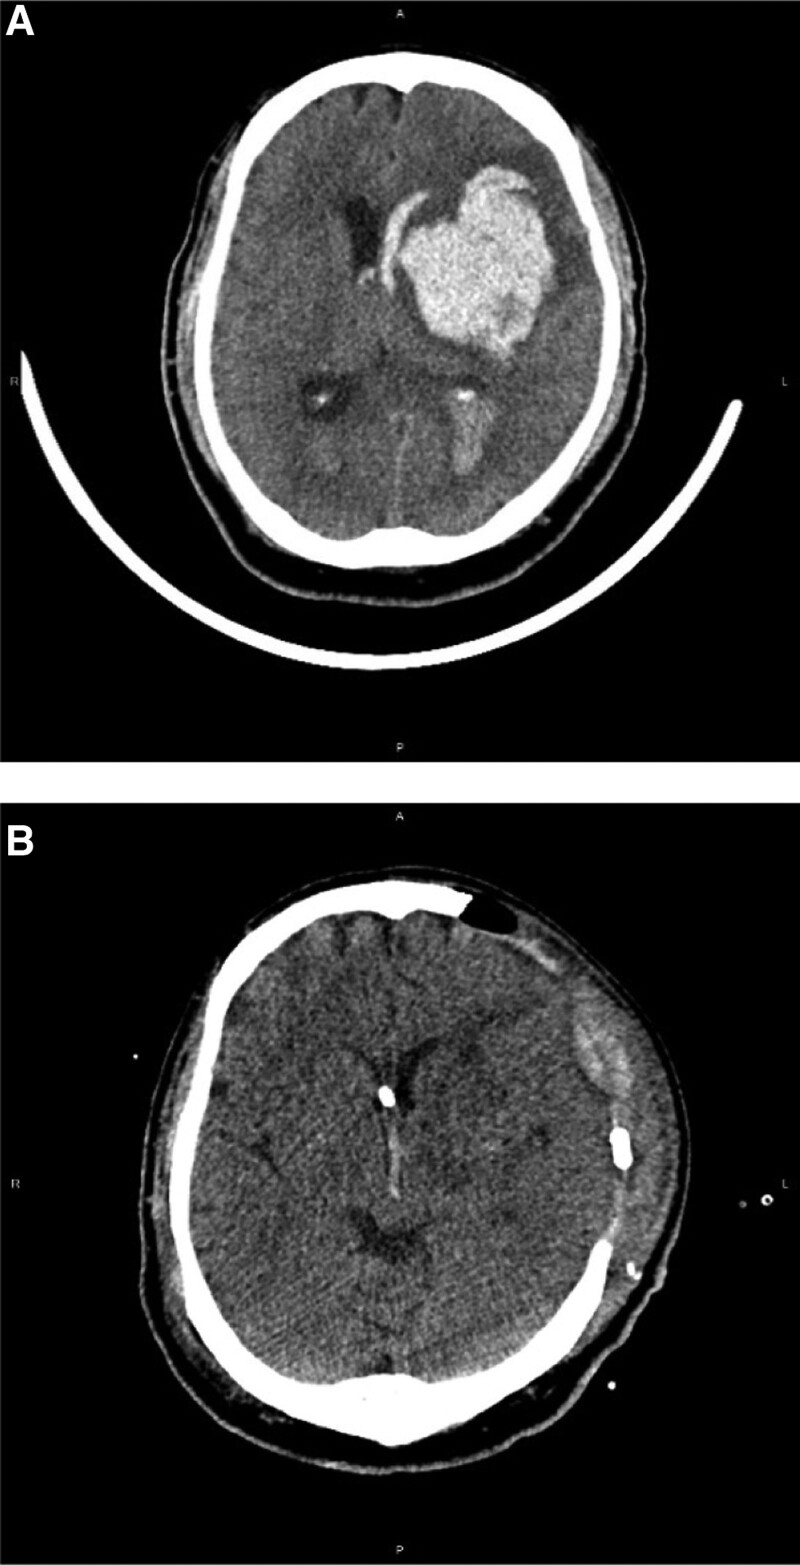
(A) The 20^th^ patient in our study, is a 66-year-old male, had a GCS of E2M4V2 when he was arrived to hospital. He suffered from spontaneous ICH with brain stem herniation over the left basal ganglion and intraventricular hemorrhage. (B) He received decompressive craniectomy and EVD after he was transferred to our hospital or about 445 min later. Brain CT scan on the 7^th^ day after operation showed nearly complete hematoma evacuation and no hydrocephalus after we clamped the EVD. We then removed the EVD on the 13^th^ day after operation. The GCS showed E3M6VT (T: tracheostomy) when he was transferred to the ordinary ward; the patient didn’t have symptoms of hydrocephalus on further follow up. CT = computerized tomography, EVD = external ventricular drainage, ICH = intracranial hemorrhage, GCS = Glasgow Coma Scale.
